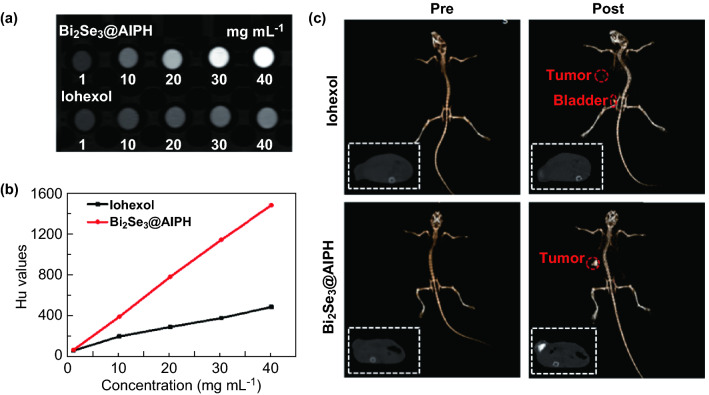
In vitro and in vivo CT imaging. a The in vitro CT imaging pictures and b quantitative HU values of Bi_2_Se_3_@AIPH NPs and iohexol at the concentrations of 1, 10, 20, 30, and 40 mg mL^−1^. c The 2D sectional imaging and 3D reconstruction pictures before and after the injection of Bi_2_Se_3_@AIPH NPs and iohexol
